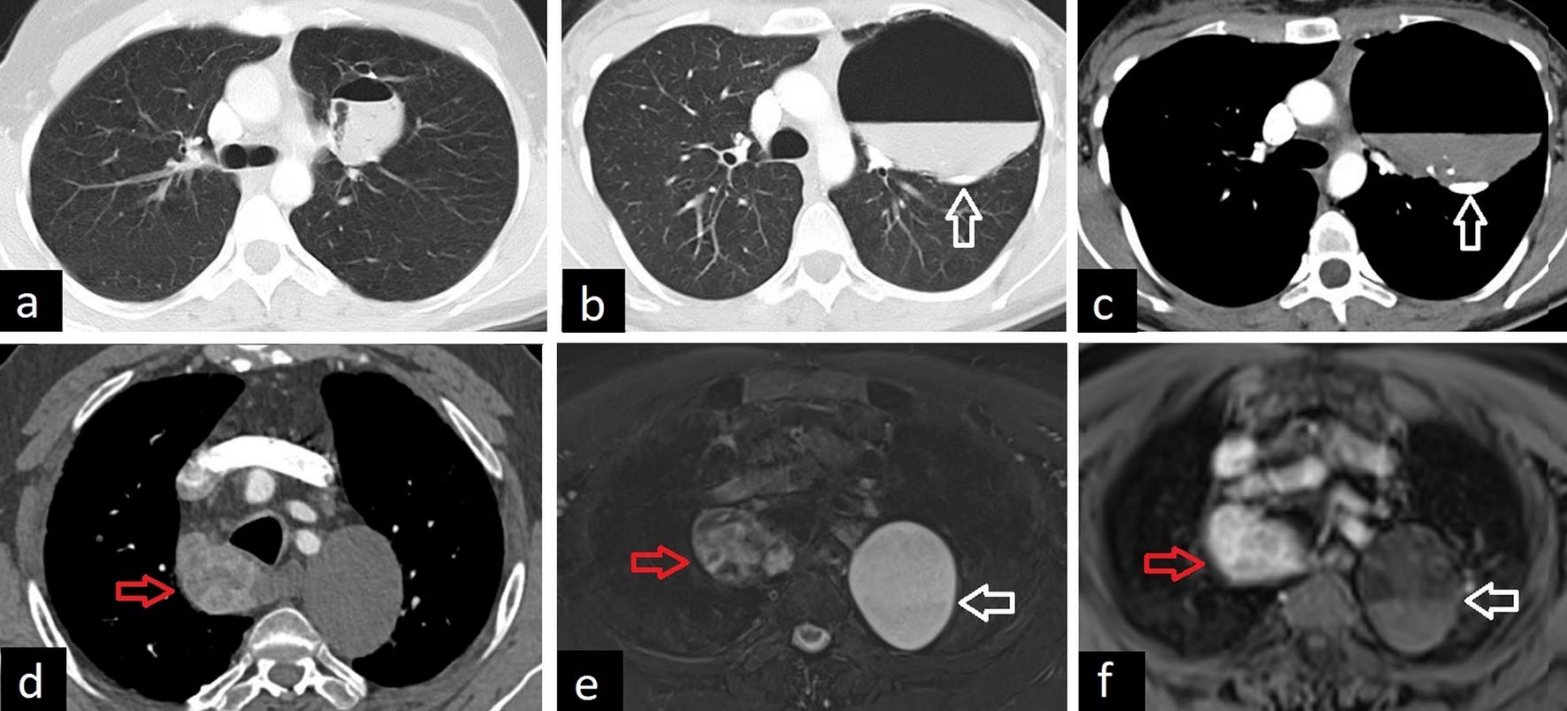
Bronchogenic cysts showing air-fluid and fluid–fluid levels. Axial CT image in lung window in a patient shows air-fluid level within the bronchogenic cyst (a). In another patient, air-fluid level and also milk of calcium can be seen within the bronchogenic cyst on axial CT images in lung (b, arrow) and mediastinal windows (c, arrow). Axial CT image in mediastinal window in another patient shows a bronchogenic cyst (d, white arrow). Fat suppressed T2 weighted (e, white arrow) and post-contrast T1 weighted (f, white arrow) MR images demonstrate the fluid-fluid level within the cyst. The red arrows show the ectopic thyroid nodule (d–f)
